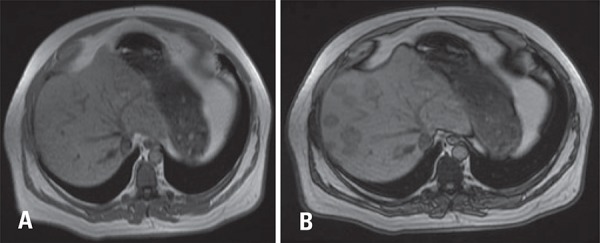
Magnetic resonance imaging in gradient echo sequences. (A) In-phase and out-of-phase images. (B) Show signal drop on the latter, indicating intracellular lipid content
